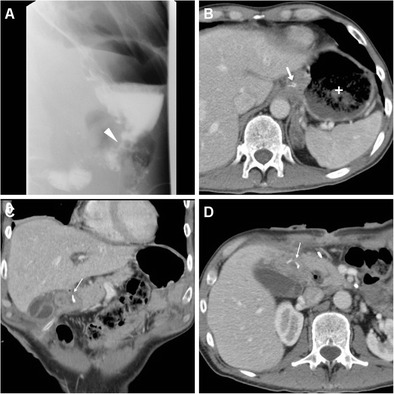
A 63-year-old male underwent partial gastrectomy with Billroth II reconstruction to relieve a large, non-malignant pyloric ulcer refractory to medical therapy. Early postsurgical CF (A) showed mildly delayed transit of iodinated contrast through the GJS (arrowhead), with fluid level in the gastric remnant. Corresponding CT (B-D) appearances before discharge included dilated remnant stomach (+) with ingested material, surgical staples at the gastric resection site (arrow in B), expected imaging appearance of the duodenal stump (DS, thin arrows in C, D) indicated by a single metallic staple, without surrounding fluid or collections
